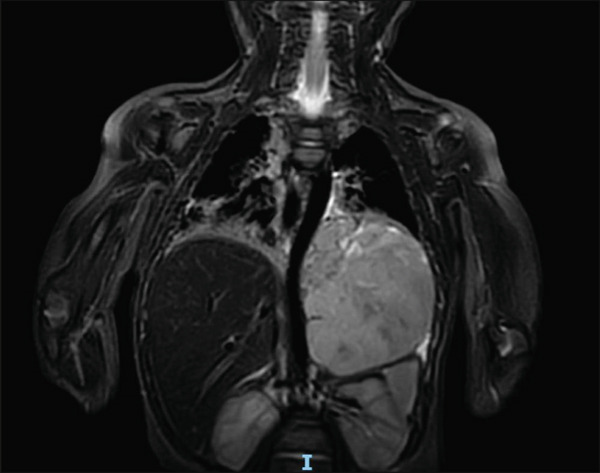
Magnetic resonance imaging (MRI) revealing large heterogeneous fairly‐well encapsulated lobulated enhancing left intrathoracic vs. suprarenal solid mass measuring approximately 6.0 × 6.9 × 8.2 cm.
